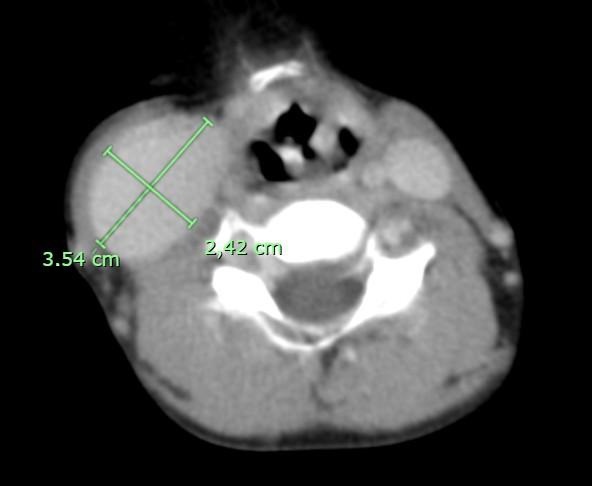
CT image in axial plane with intravenous contrast, showing the right internal jugular vein.
